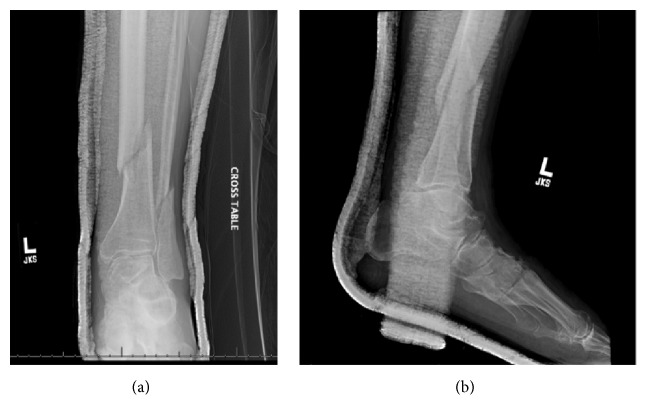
Preoperative AP and lateral radiographic images of the left leg.
